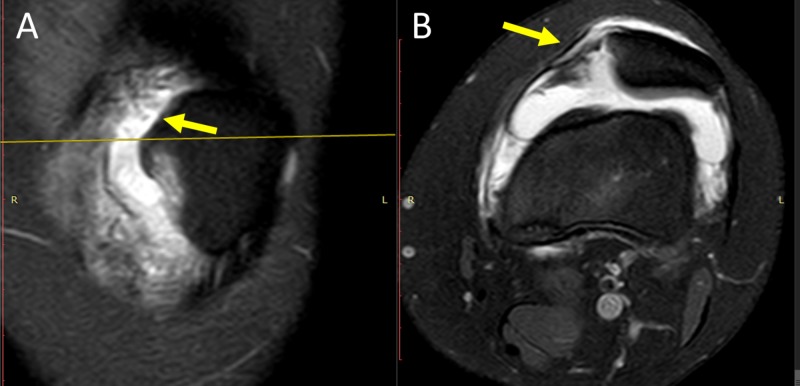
MRI of left Knee: patellar attachment of MPFL A (frontal plane): the arrow indicates the proximal part of the medial patellar border. B (axial plane): the arrow indicates continuous MPFL MRI: magnetic resonance imaging; MPFL: medial patellofemoral ligament
